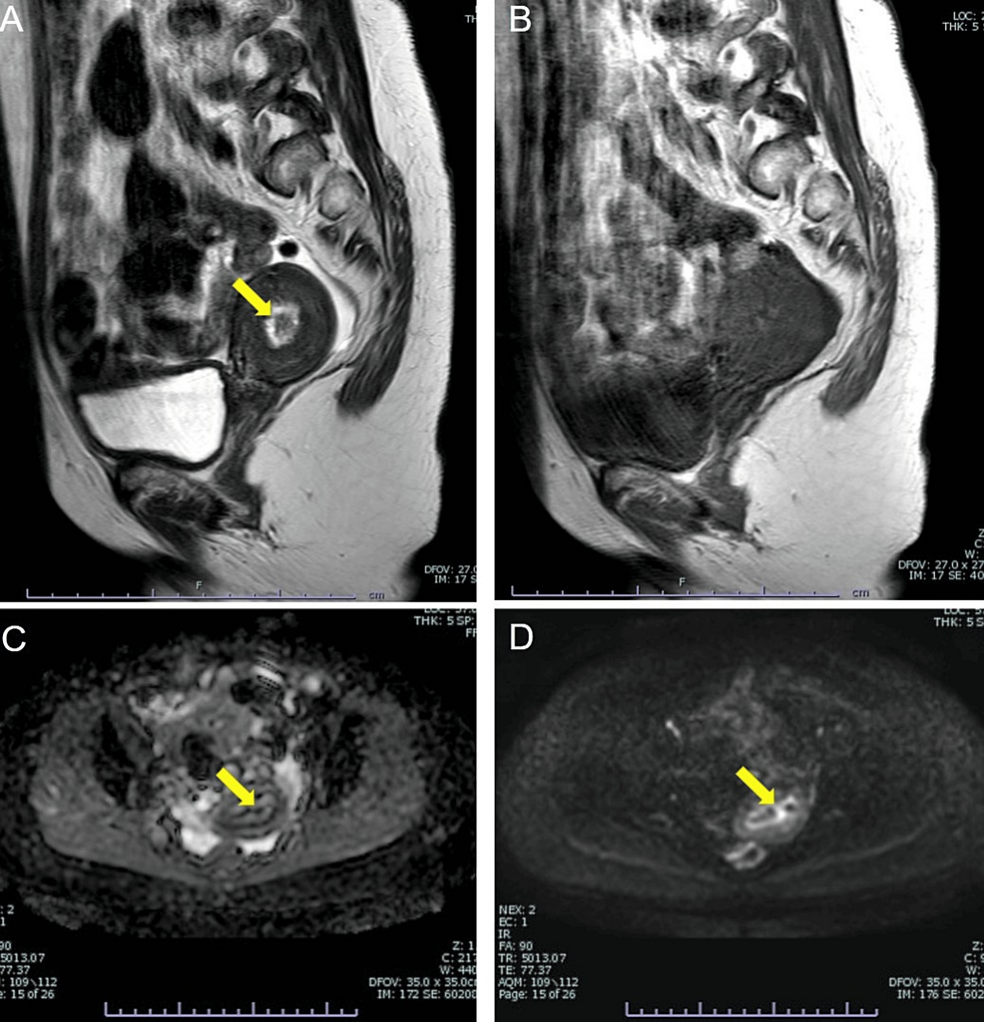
Magnetic resonance imaging (MRI) scans of a uterine tumor. T2-weighted image (T2WI) showing an 11-mm-sized tumor in the uterine cavity with isointensity. (A) The yellow arrow indicates the tumor. T1-weighted image of the same slice as in T2WI, showing the tumor. (B) Diffusion-weighted image (DWI) showing high intensity in the tumor, indicated by the yellow arrow (C). (D) Apparent diffusion coefficient (ADC) map showing low intensity in the tumor, indicated by the yellow arrow.
